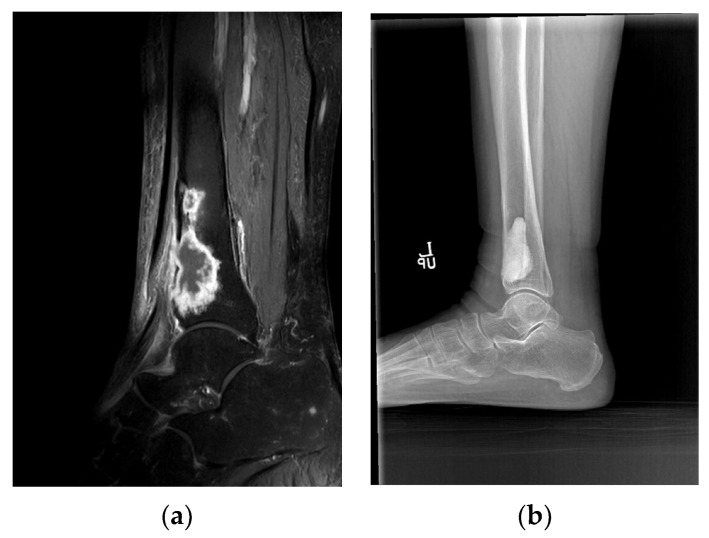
Antibiotic-mixed cement filling in a 75-year-old female patient (identified pathogen: Mycobacterium tuberculosis). (a) Sagittal MRI shows chronic osteomyelitis on the distal tibia shaft preoperatively. (b) Lateral radiograph shows that antibiotic-impregnated cement filling is well maintained 2 years postoperatively.
